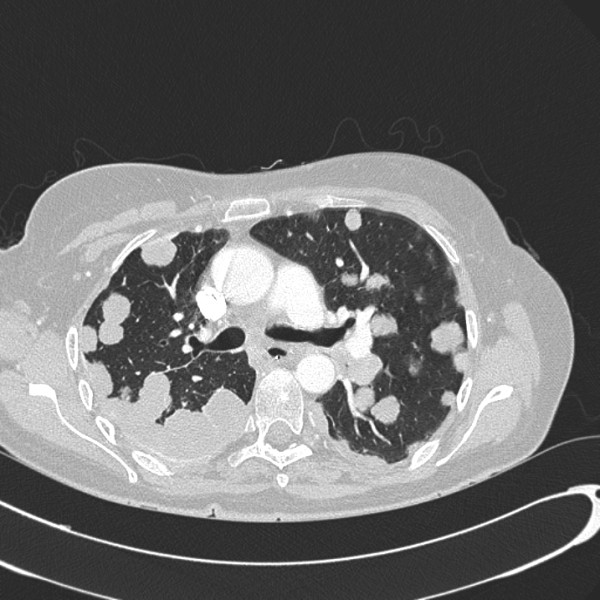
Distant metastasis-axial chest CT showing tumour metastasis to the plural spaces and parenchyma of the lungs.
